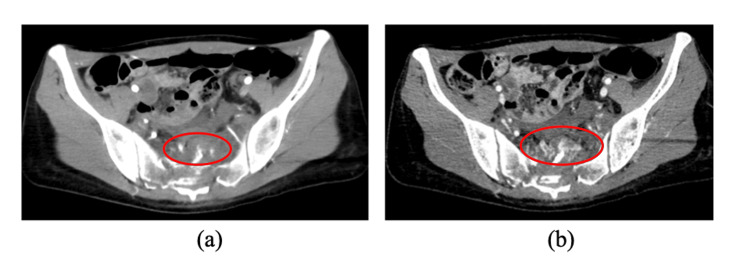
Initial contrast-enhanced computed tomography (a) Arterial phase: Active extravasation of contrast is observed on the anterior surface of the sacrum (red circle). (b) Venous phase: An increase in contrast accumulation is noted (red circle).
